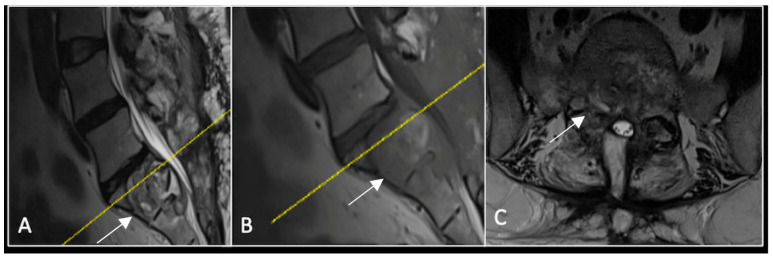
MRI imaging following separation surgery. (A) T2W sagittal; (B) T1W sagittal; (C) T2W axial. Imaging shows removal of posterior lumbosacral elements with residual disease (arrow) within the S1 vertebral body but significant reduction in tumour dimensions. The yellow line on the sagittal images indicates the level at which the axial image was obtained.
